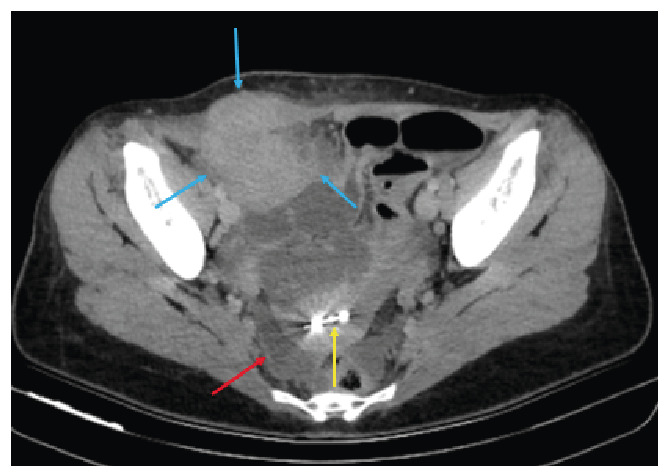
Axial pelvic CT demonstrates an abdominal wall mass (blue arrows) extending into the adjacent intra-abdominal and subcutaneous tissues, accompanied by pelvic fluid accumulation (red arrow). An intrauterine device (IUD) is seen inside the uterine cavity (yellow arrow).
